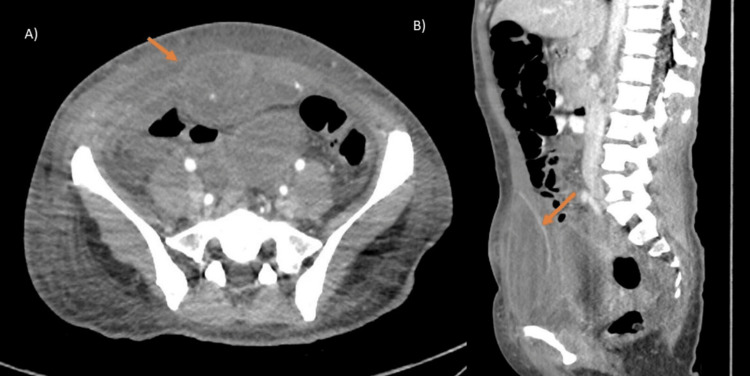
Arterial phase abdominopelvic CT scan: (A) axial view and (B) sagittal view A well-defined oval lesion (arrow) measuring 5×7 cm is observed in the hypogastric region at the right rectus abdominis, with fluid attenuation and peripheral enhancement after contrast administration. No additional abdominal lesions are identified. CT: computed tomography
